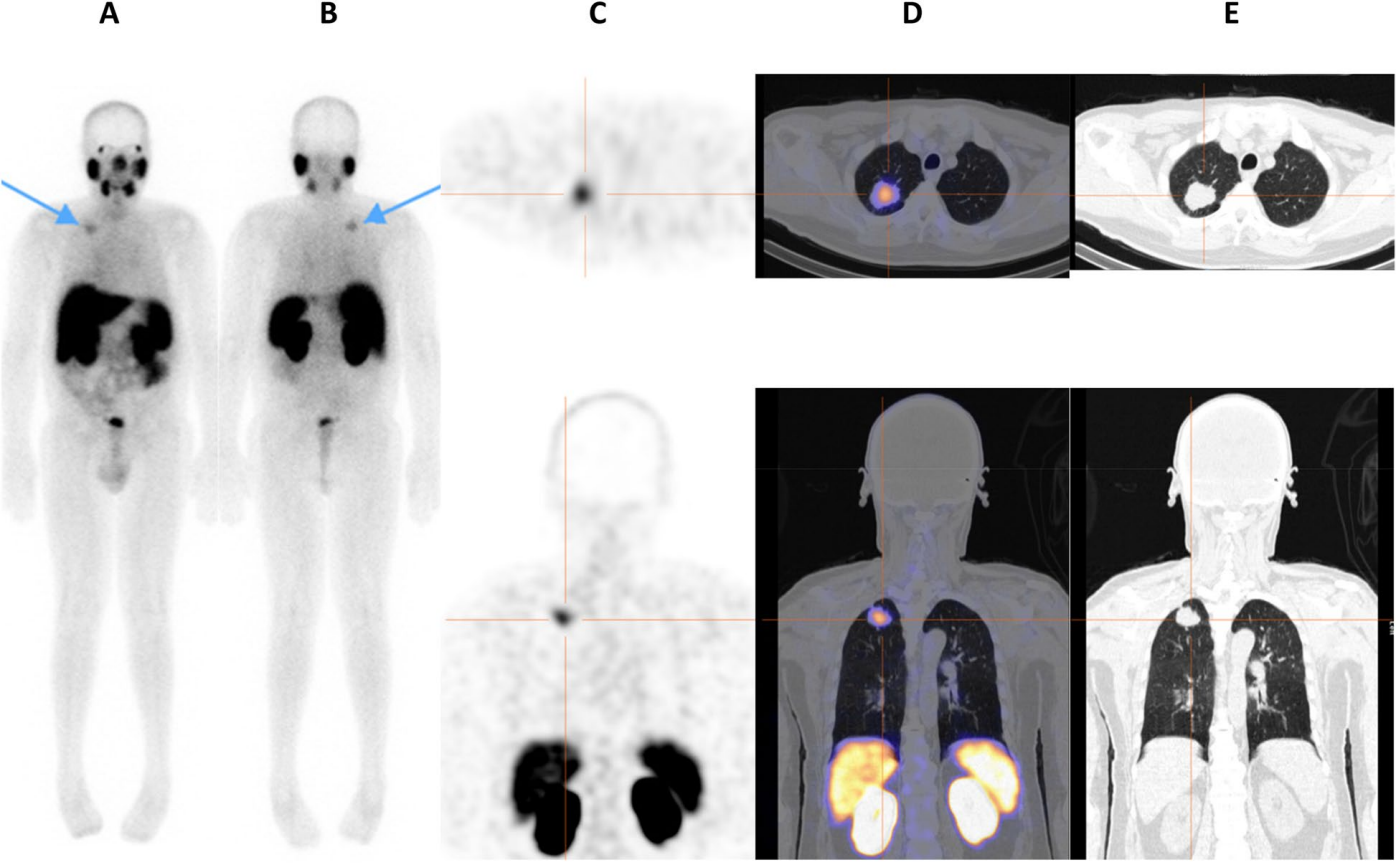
PSMA avid lesion was incorrectly reported as a rib metastasis on planar imaging (A & B- tip of arrows on anterior and posterior projections) and as a lung metastasis on SPECT images (C - crosshairs on trans-axial and coronal views). Right lung lesion on SPECT/CT (D) with corresponding CT findings (E) were suspicious for a second primary malignancy. Biopsy confirmed a primary lung adenocarcinoma
